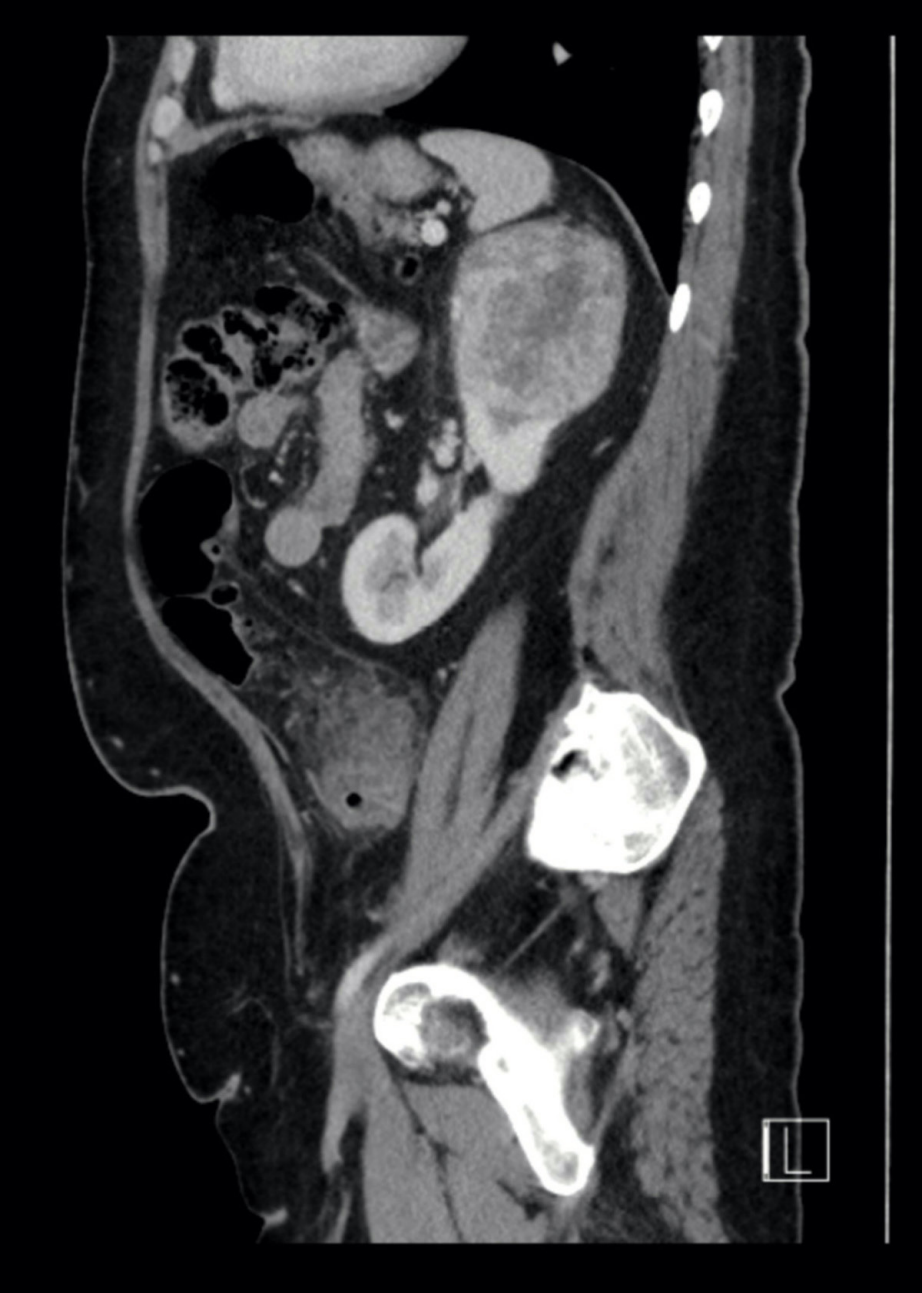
Sagittal CT view showing upper pole right renal tumor.
